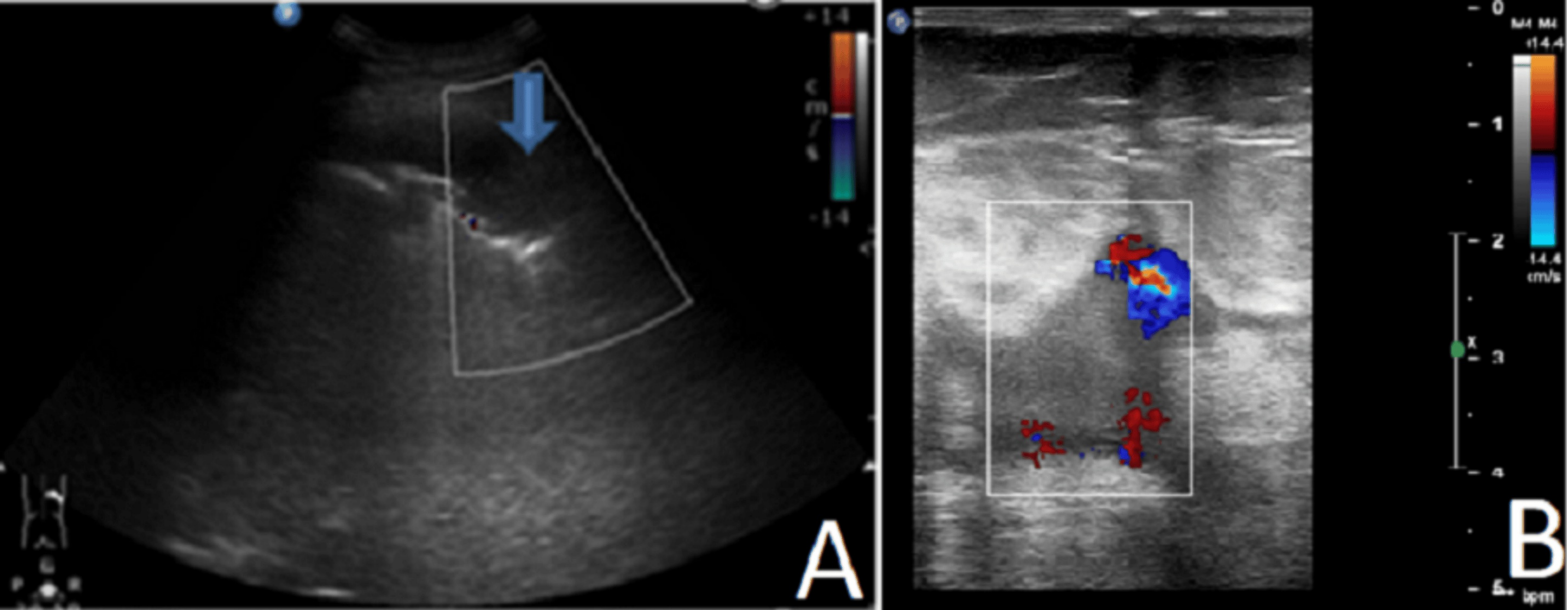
(A) Chest ultrasound. Subpleural consolidation measuring 25/45 mm, air bronchogram, in the absence of CD vascular signals. (B) Compressive and CD ultrasound of the lower extremities. Thrombosis of the right common femoral vein in the area of ​​insertion of the VSM dex. Abbreviation: CD: Color Doppler
